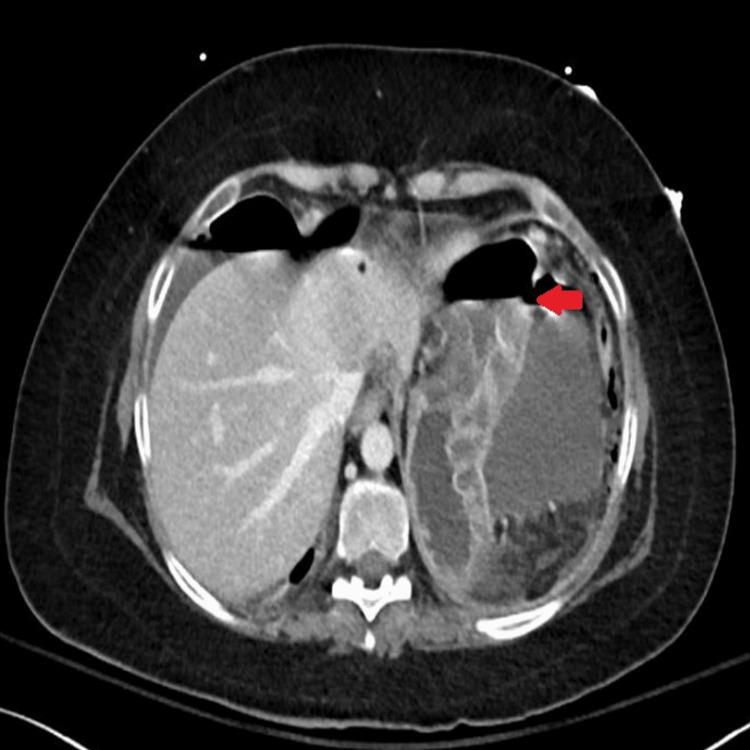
Horizontal-section CT image showing features of gastric perforation likely related to the underlying perforated peptic ulcer with 0.8 cm defect at the distal greater curvature
